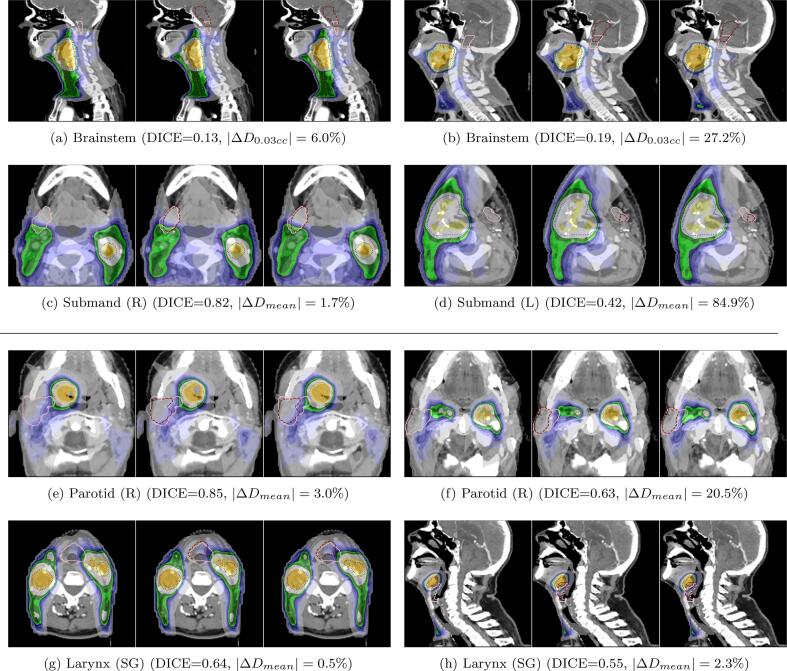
CT scans of photon (a–d) and proton (e–h) patients overlayed with a dose distribution as well as PTV (DL1) (orange), PTV (DL2) (blue), manual (pink) and automated (maroon) contours. Each example shows the $P_{\mathrm{OG}},P_{\mathrm{MC}}$ and $P_{\mathrm{AC}}$ plans from left to right. The dose metric in the sub-captions compares the absolute percentage difference of $P_{\mathrm{MC}}-P_{\mathrm{AC}}$. (For interpretation of the references to colour in this figure legend, the reader is referred to the web version of this article.)
